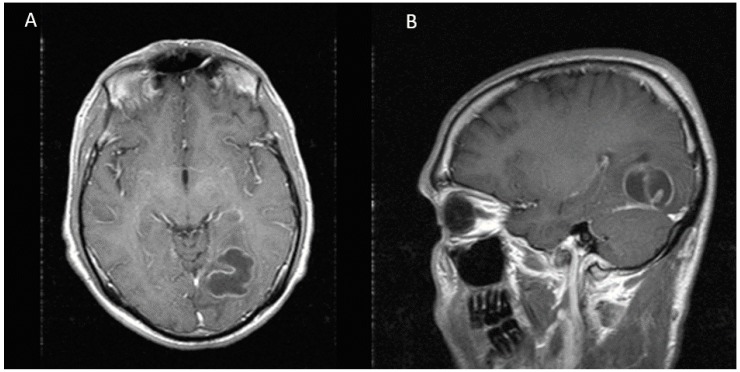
MRI showing a multilobulated and multiseptated lesion of 31 × 31 × 26 mm with peripheral enhancement in the left occipital lobe. (A) Axial view (B) Sagittal view.
